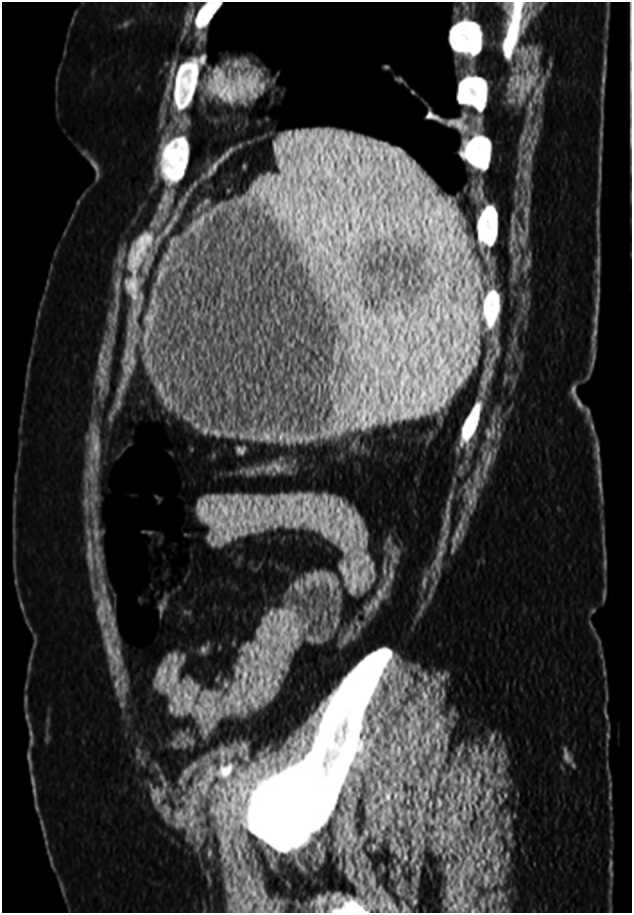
Sagittal CT scan showing massive splenomegaly and splenic hypodense lesions.
